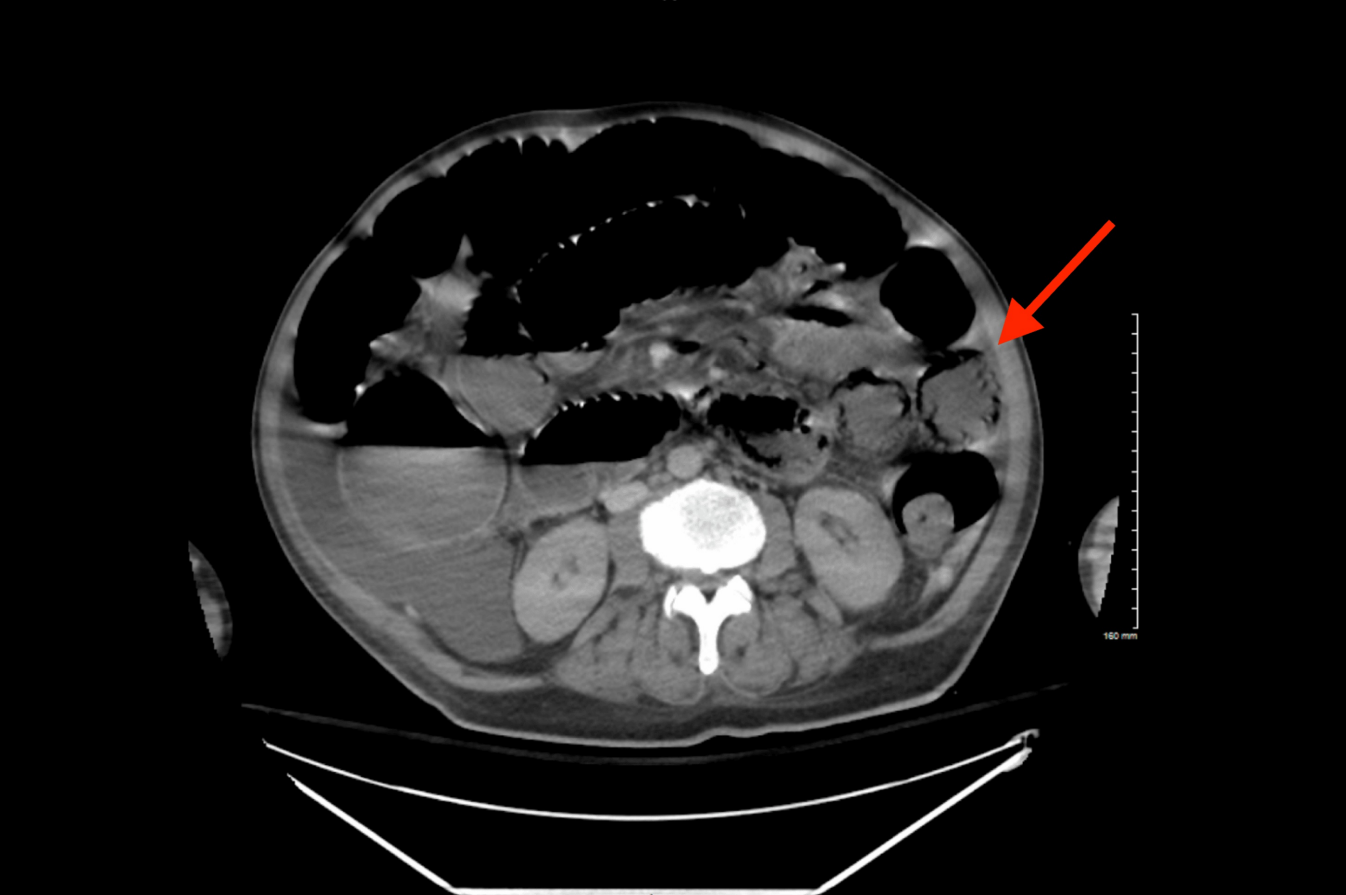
CT abdomen and pelvis with IV contrast axial view demonstrating pneumatosis involving multiple loops of the mid and distal small bowel (red arrow)
